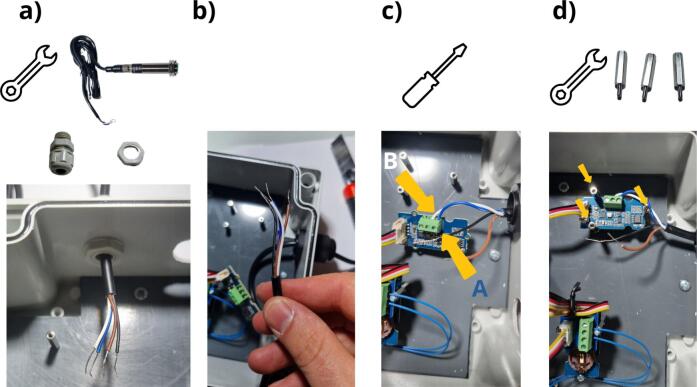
Installation of the Ultrasonic Sensor URM14 SEN0358: a) attaching the gland and installing the sensor wire, b) presenting the wires, c) connecting the RS485 module, d) attaching the RS485 module to the housing.
